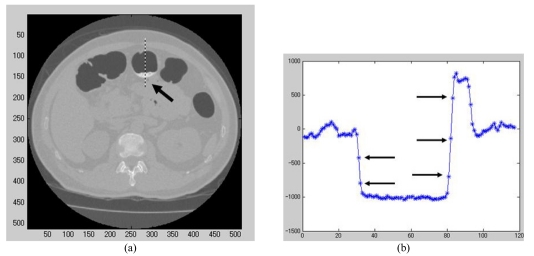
Pixels influenced by partial volume effect. (a) An axial CT image obtained during CTC before EC. Tool tagging was shown in a high intensity part (arrow). (b) Profile plot at the border of air and tagged material. A few pixels influenced by partial volume effect (arrow).
